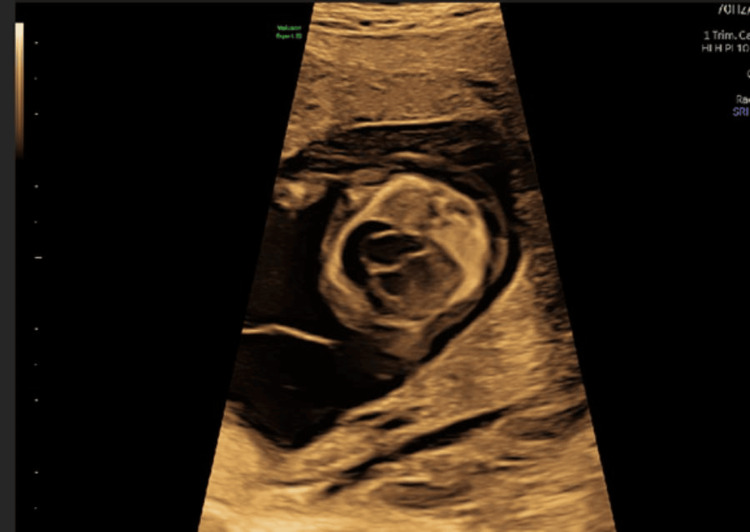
First-trimester ultrasound demonstrating cardiomegaly and cardiac malformation that is difficult to characterize, and pericardial effusion.
